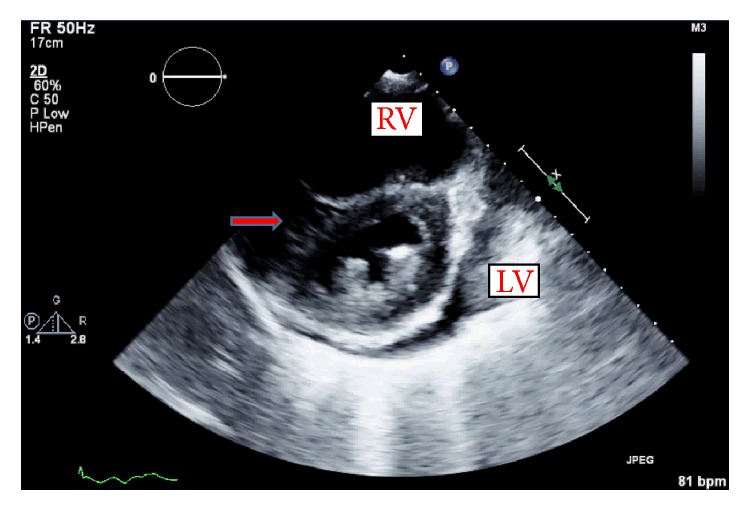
Transthoracic echocardiogram 2D during end-diastolic phase, after hemodialysis showing improvement of flattening (red arrow) of the interventricular septum (D-shaped left ventricle) and displacement toward left ventricle (LV). Right ventricle (RV).
